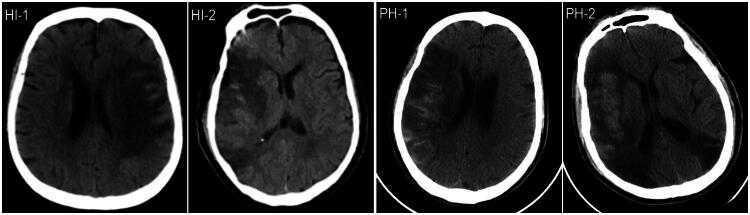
Different types of acute ischemic stroke haemorrhagic transformation confirmed by brain CT.
